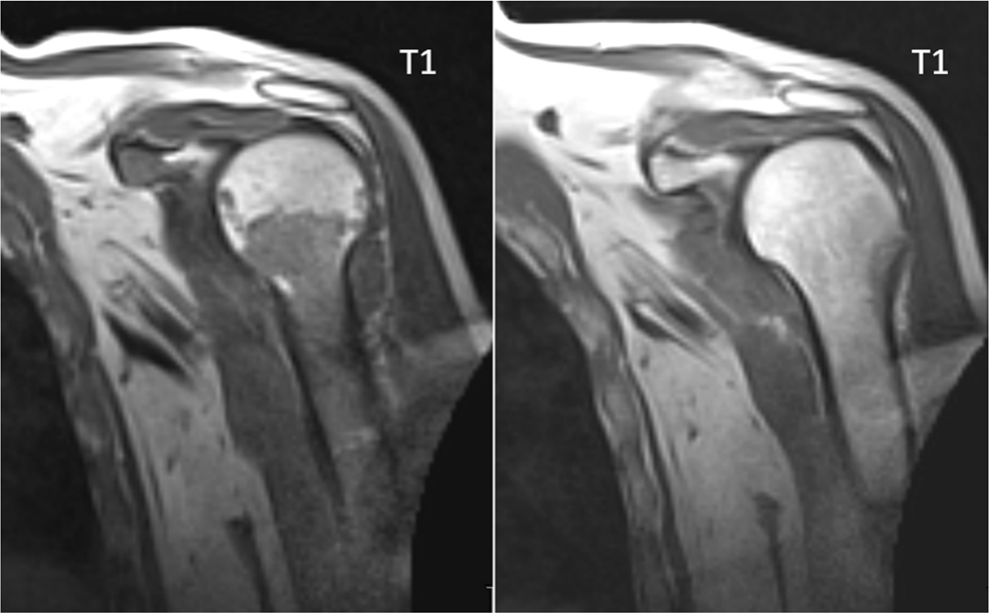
T1-weighted image of the humerus before (*left*) and after (*right*) systemic therapy. Remark the progressive fading of the bone marrow signal intensity, from hypo-intense to hyperintense signal intensity on T1-weighted imaging, indicating response to therapy
